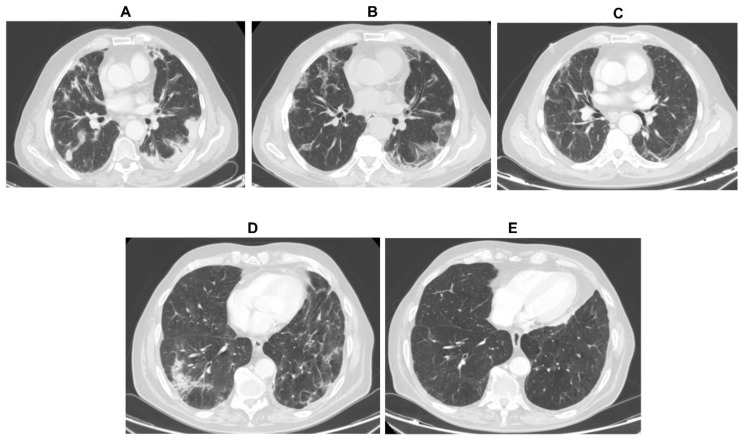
Computed tomography (CT) scan imaging. Panel (A–C): 80-year-old male with metastatic non-small-cell lung cancer (mNSCLC) showing a classical cryptogenic organized pneumonia pattern. (A) Axial chest CT image obtained after 10 months of treatment with nivolumab showing multiple consolidations involving both lungs. (B) Axial follow-up CT-image obtained 1 month later showing an improvement of pneumonitis and the presence of ground-glass opacities. (C) Axial follow-up CT image obtained 11 months later showing pneumonitis resolution and the presence of bilateral fibrotic alteration. Panel (D–E): 70-six-year-old male with mNSCLC presenting a not otherwise specified pattern of pneumonitis. (D) Axial chest CT image obtained 2 months after the beginning of the treatment with nivolumab showing multiple consolidations, interlobular septal thickening, subpleural reticulations. (E) Axial chest CT image obtained 8 months later showing a complete resolution of the signs.
